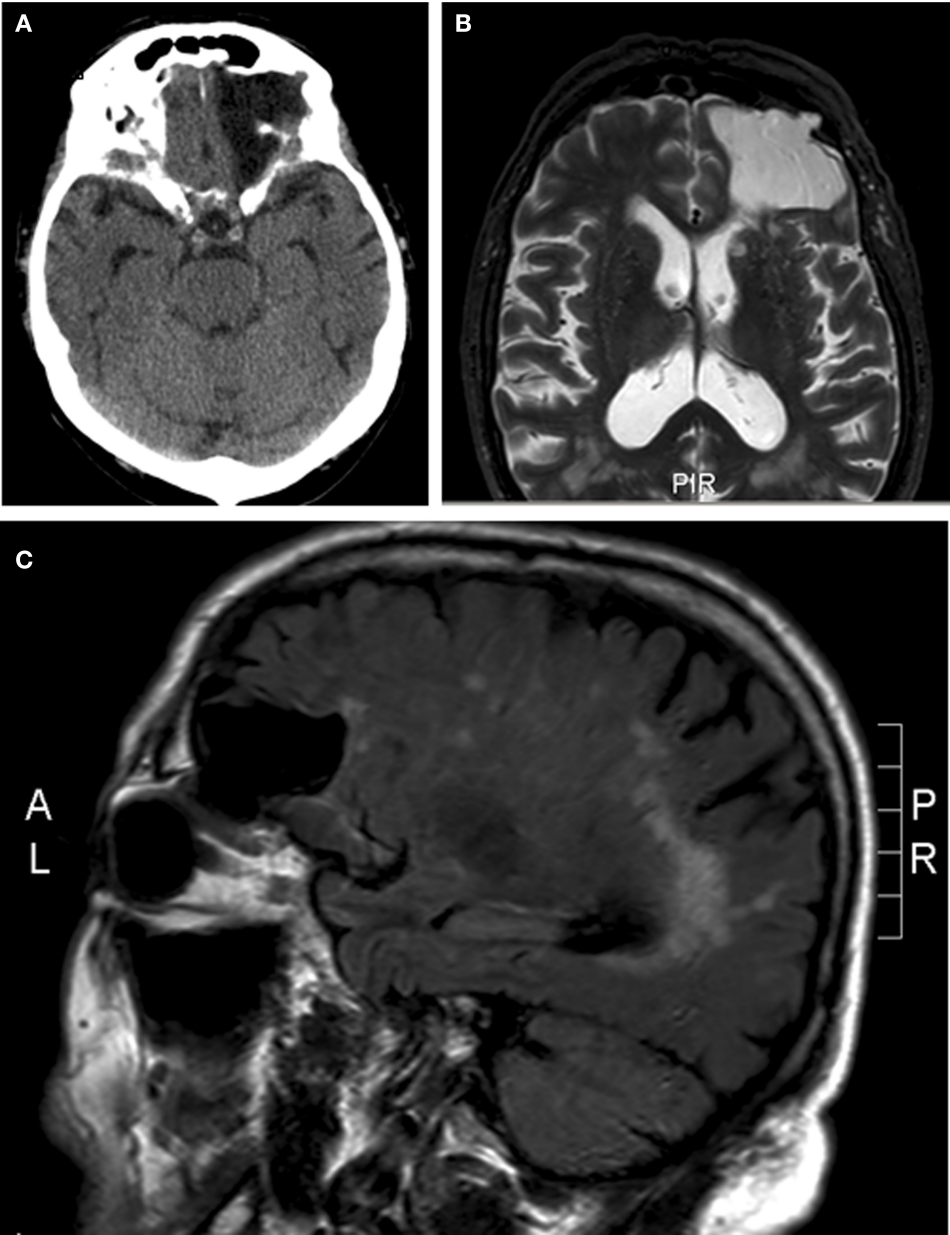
Meningocele into the left orbit due to a bony defect in the orbital roof. (A) Axial computed tomography. (B) Axial magnetic resonance imaging short T1 inversion recovery sequence. (C) Sagittal magnetic resonance imaging, fluid-attenuated inversion recovery sequence.
